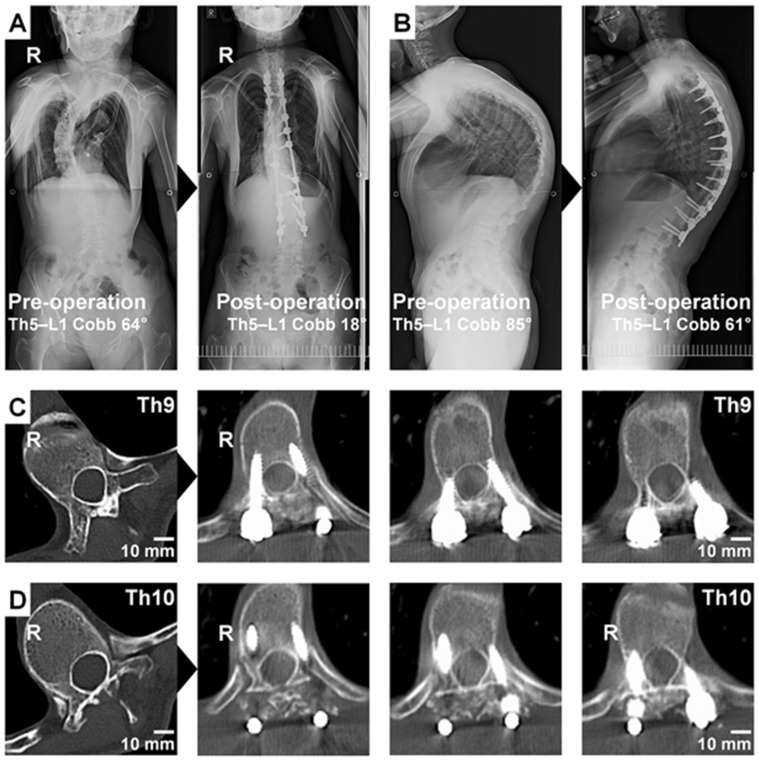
A technical note of a probe with an electrical conductivity-measuring device (ECD) in a 20-year-old man with the Sotos syndrome and scoliosis. (A,B) Anteroposterior (A) and lateral (B) whole spine radiographs at pre-operation and post-operation. His right-curved scoliosis and kyphosis at Th5–L1 were surgically treated. (C,D) Axial CT images at pre-operation and post-operation. The diameter of left concave side’s pedicles at Th9 (C) and Th10 (D), the apical vertebrae of scoliosis, was severely reduced because of the deformity. The ECD probe was useful to place pedicle screws safely without perforation of the medial wall of left Th9 and Th10 pedicles. Consequently, in-out-in pedicle screw insertion was accomplished at the left side of both segments under the ECD guidance of audio alerts and light-emitting diode warning signals. In all images, R indicates the right side of the body.
